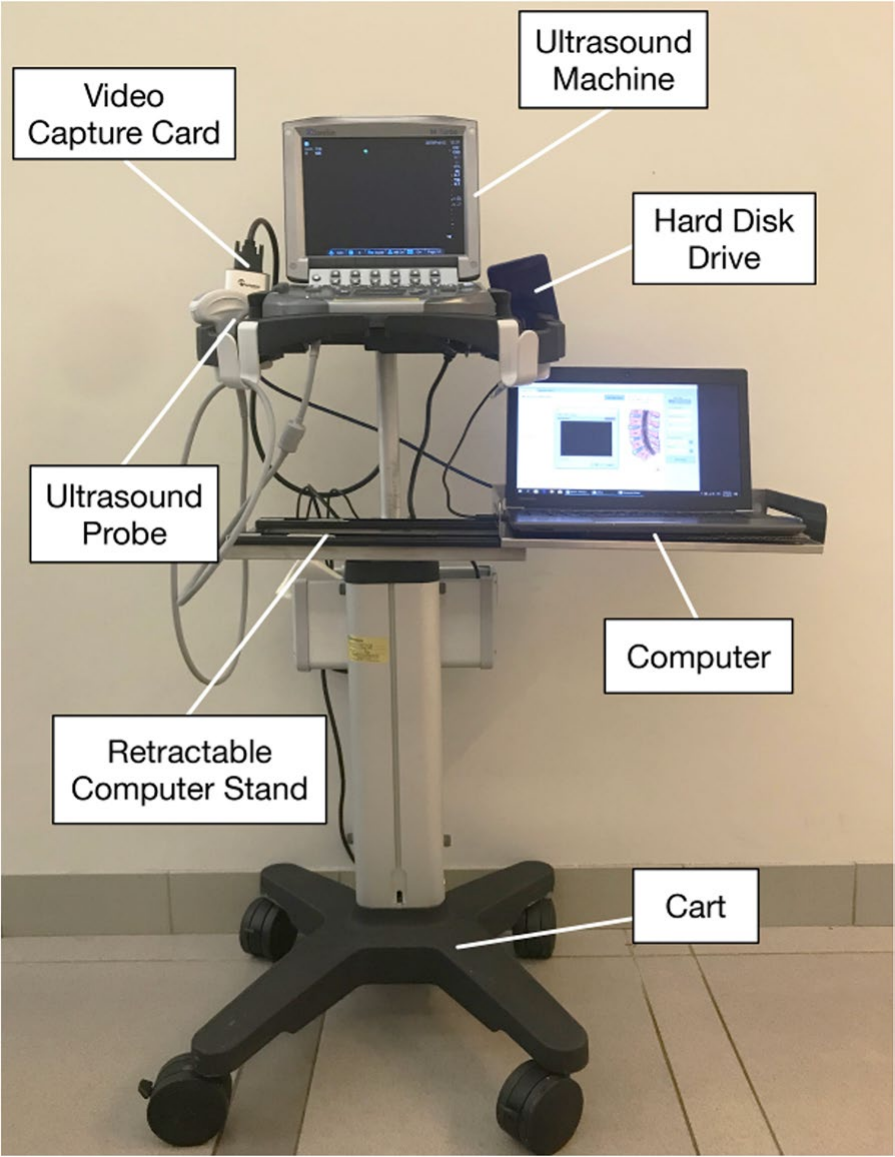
System setup of the automated ultrasound-guided spinal landmark identification program
